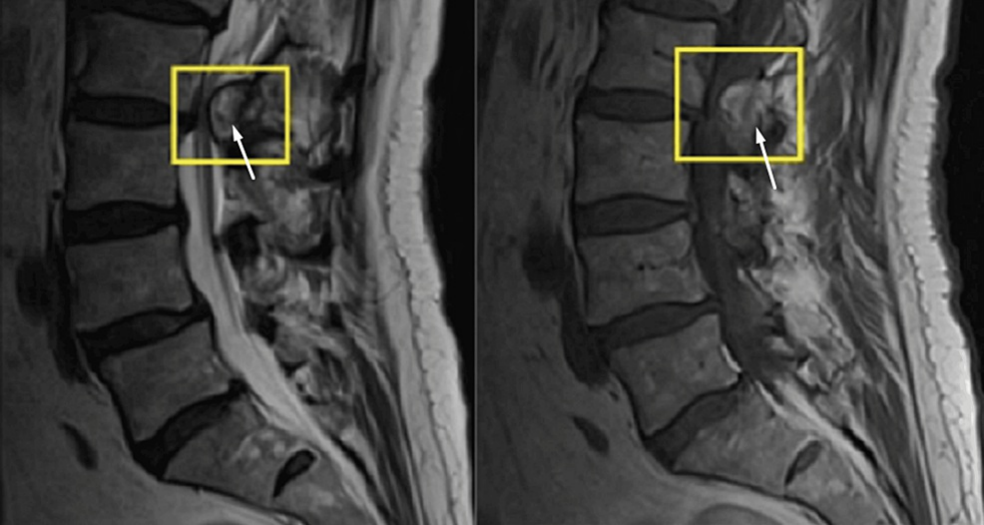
Preoperative MRI: sagittal plane The MRI found evidence of a formation in the L2-L3 epidural space compressing the nerve structures MRI: magnetic resonance imaging
